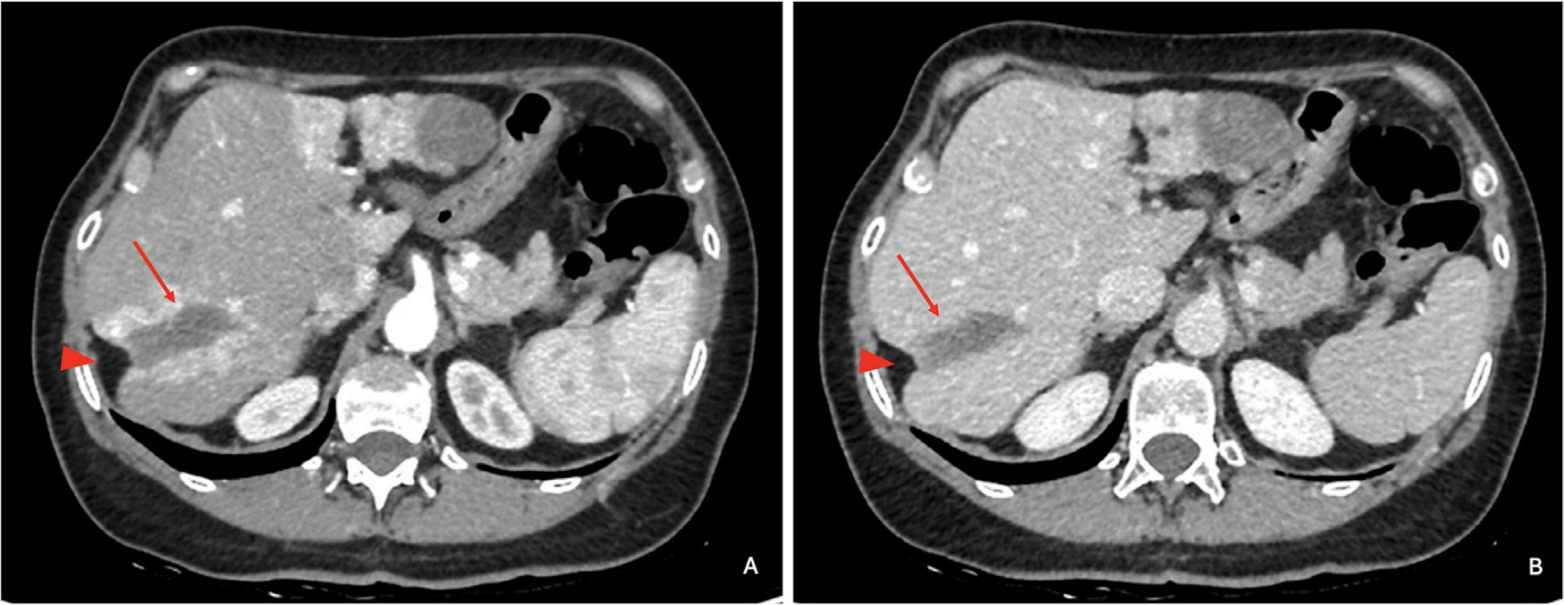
Computed tomography images. An oblong formation at the VII hepatic segment (arrow), measuring 50 × 20 mm, is observed to cause distortion of the capsular profile (arrow head) in both the arterial (A) and venous phases (B).
